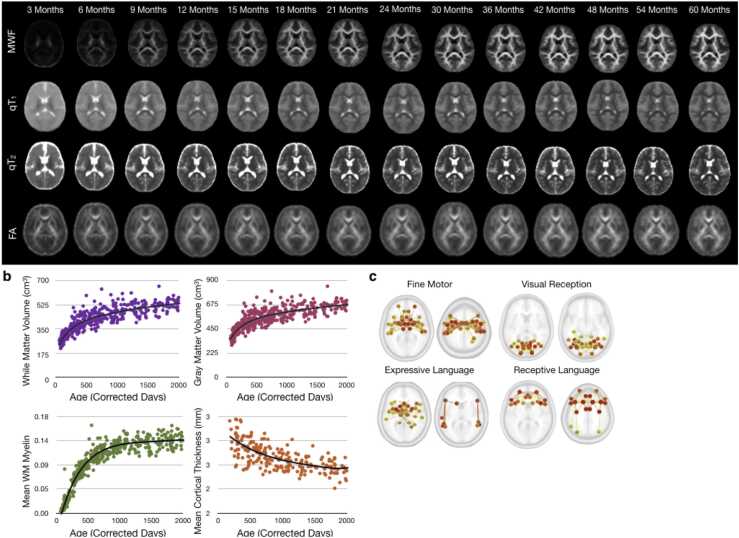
(a) General multimodal MRI patterns of brain maturation, including indices of anatomy, myelination, and functional connectivity across the first 5 years of life. (b) Curated and condensed trajectories of white and gray matter volume, myelination, and mean cortical thickness showing the changing rate of development, with most developmental processes and changes occurring rapidly over the first 1000 days and the slowing throughout the remainder of childhood. (c) Networks of functional connectivity change associated with emerging fine motor, language, and visual processing skill.
